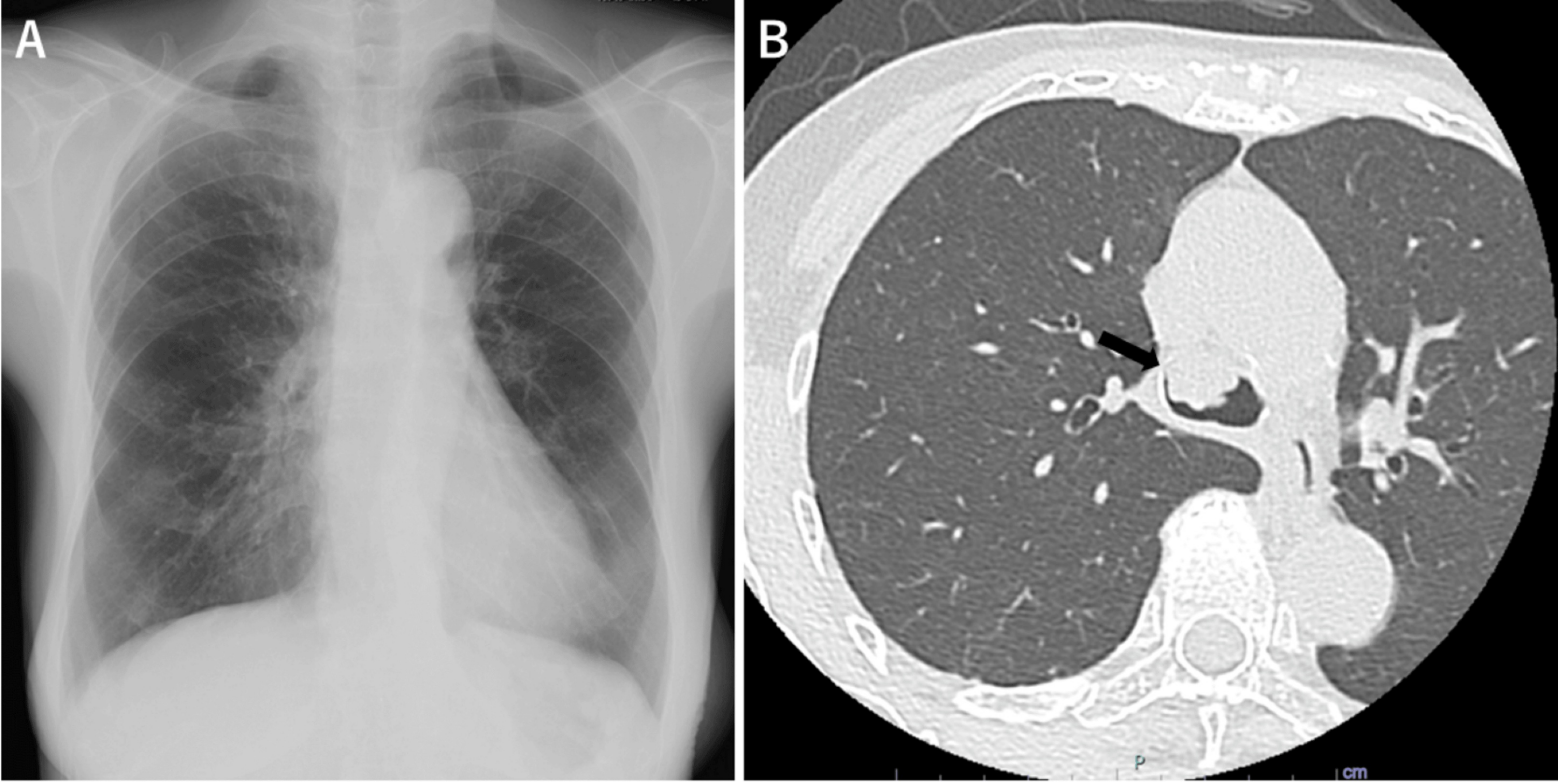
Chest images of the patient (A) Chest X-ray showing no apparent abnormalities in the lung fields or mediastinum. (B) Axial chest CT image showing an intratracheal tumor (black arrow).
